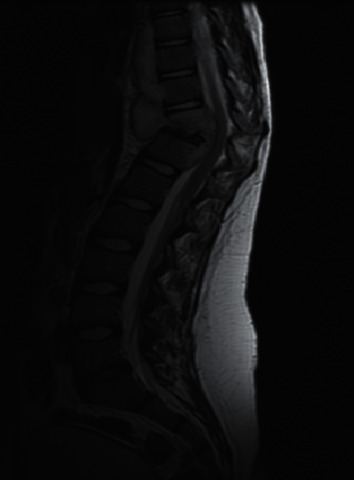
Contrasted magnetic resonance imaging (MRI) of the spine shows kyphotic destruction of thoracic vertebrae T10, T11, and T12 with abnormal high signal on T2 and low signal on T1 with heterogeneous enhancement in addition to a large heterogeneous paravertebral multiloculated prevertebral and paraspinal abscess extending from T7 through T12 with a peripheral thick wall that has low T2 and high T1 signal with intense enhancement. These are associated with an epidural collection and retropulsed collapsed T11, which result in complete attenuation of the anterior subarachnoid spaces and compression of the distal cord.
